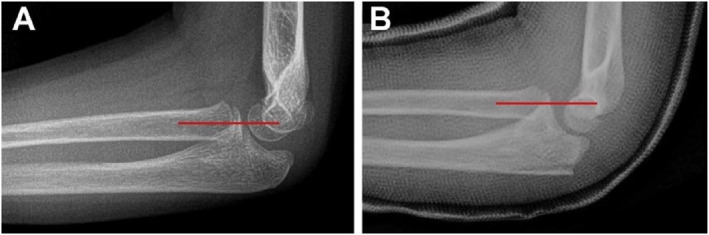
Example of a radiocoronoid line. (A) Lateral radiograph of the uninjured elbow demonstrating the radiocapitellar line intersecting the capitellum. (B) Lateral radiograph of a Monteggia lesion, with the radiocapitellar line anterior to the capitellum. Reproduced from Ref. 7 with permission from ELSEVIER, copyright 2020.
